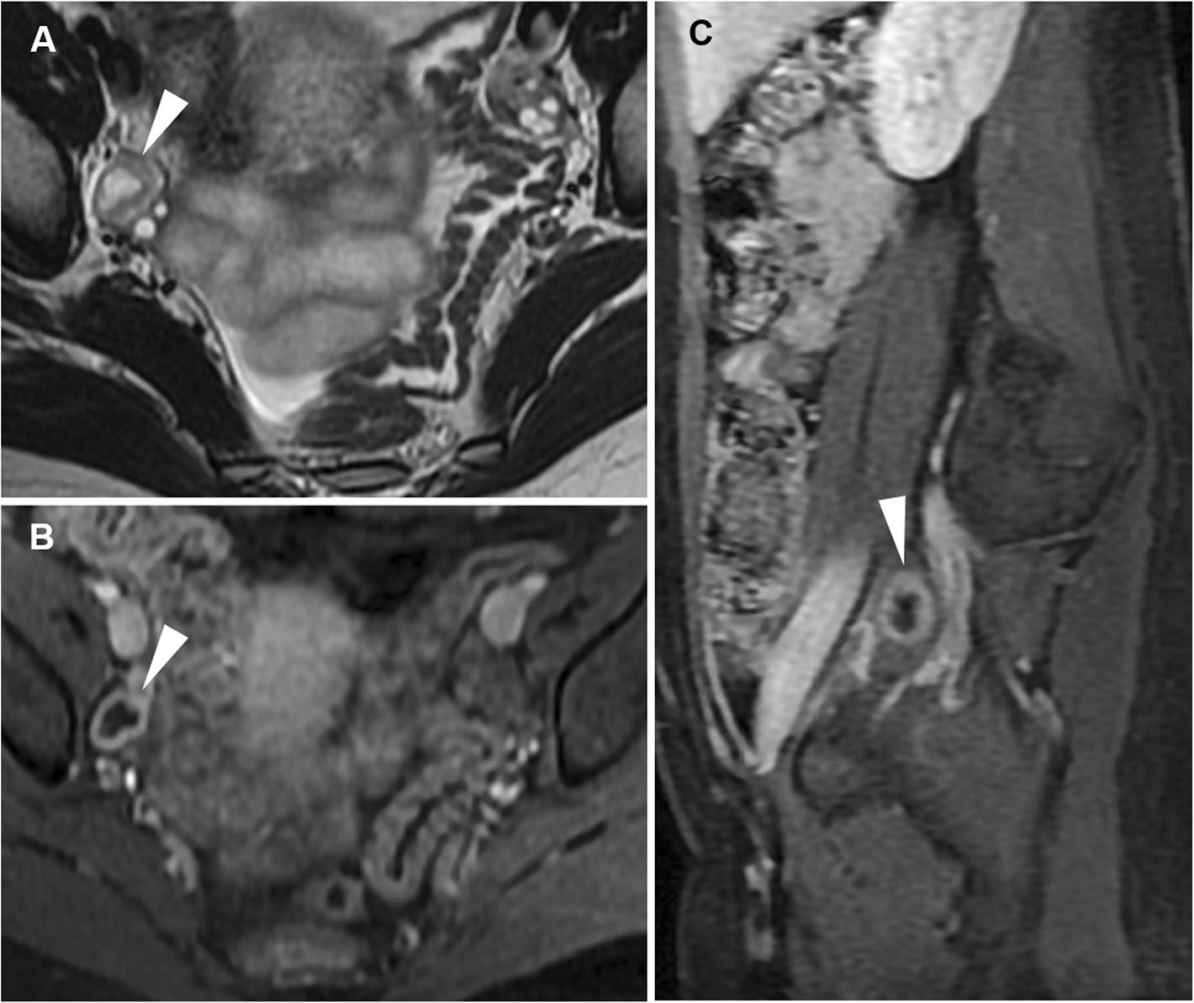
Usual MRI appearance of an uncomplicated corpus luteum in a 26-year-old woman. Oblique-coronal T2-weighted (a), oblique-coronal (b) and sagittal (c) gadolinium-enhanced fat-suppressed T1-weighted images show a small-sized, unilocular fluid-containing structure in the right ovary (arrowheads), with typical homogenous T1-hypointense signal and T2-hyperintense signal, thickened walls with a crenulated enhancing rim
